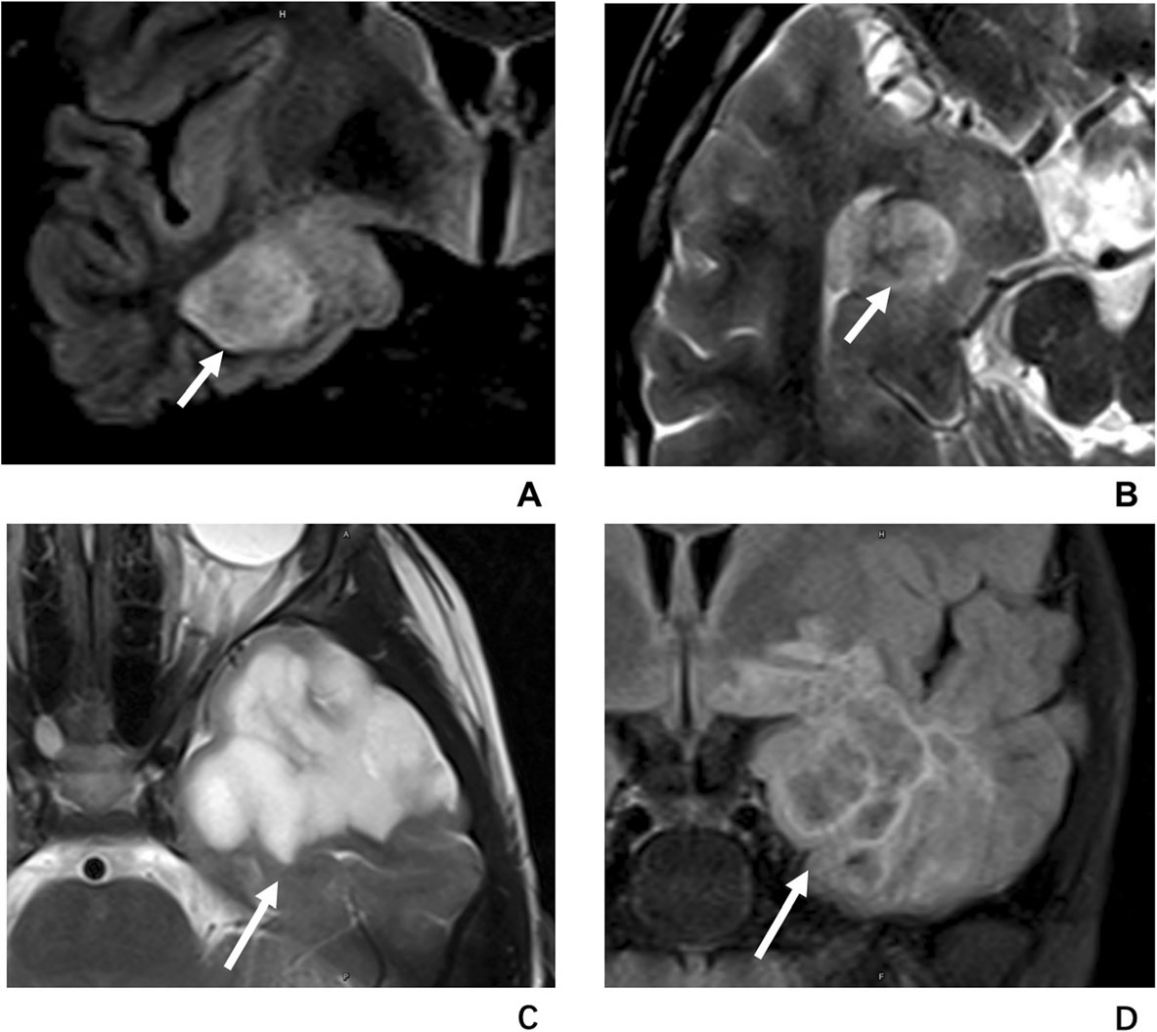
The comparison between “salt and pepper sign” in PLNTY and “soap bubble sign” in DNET. The T2WI (a) and T2-FLAIR (b) images of a case of PLNTY show the “salt and pepper sign” (short arrow) caused by grit calcification. The “soap bubble sign” (arrow) which represents the multiple cystic lesion can be seen in the T2WI (c) and T2-FLARI (d) images of a 15-year-old boy with DNET
